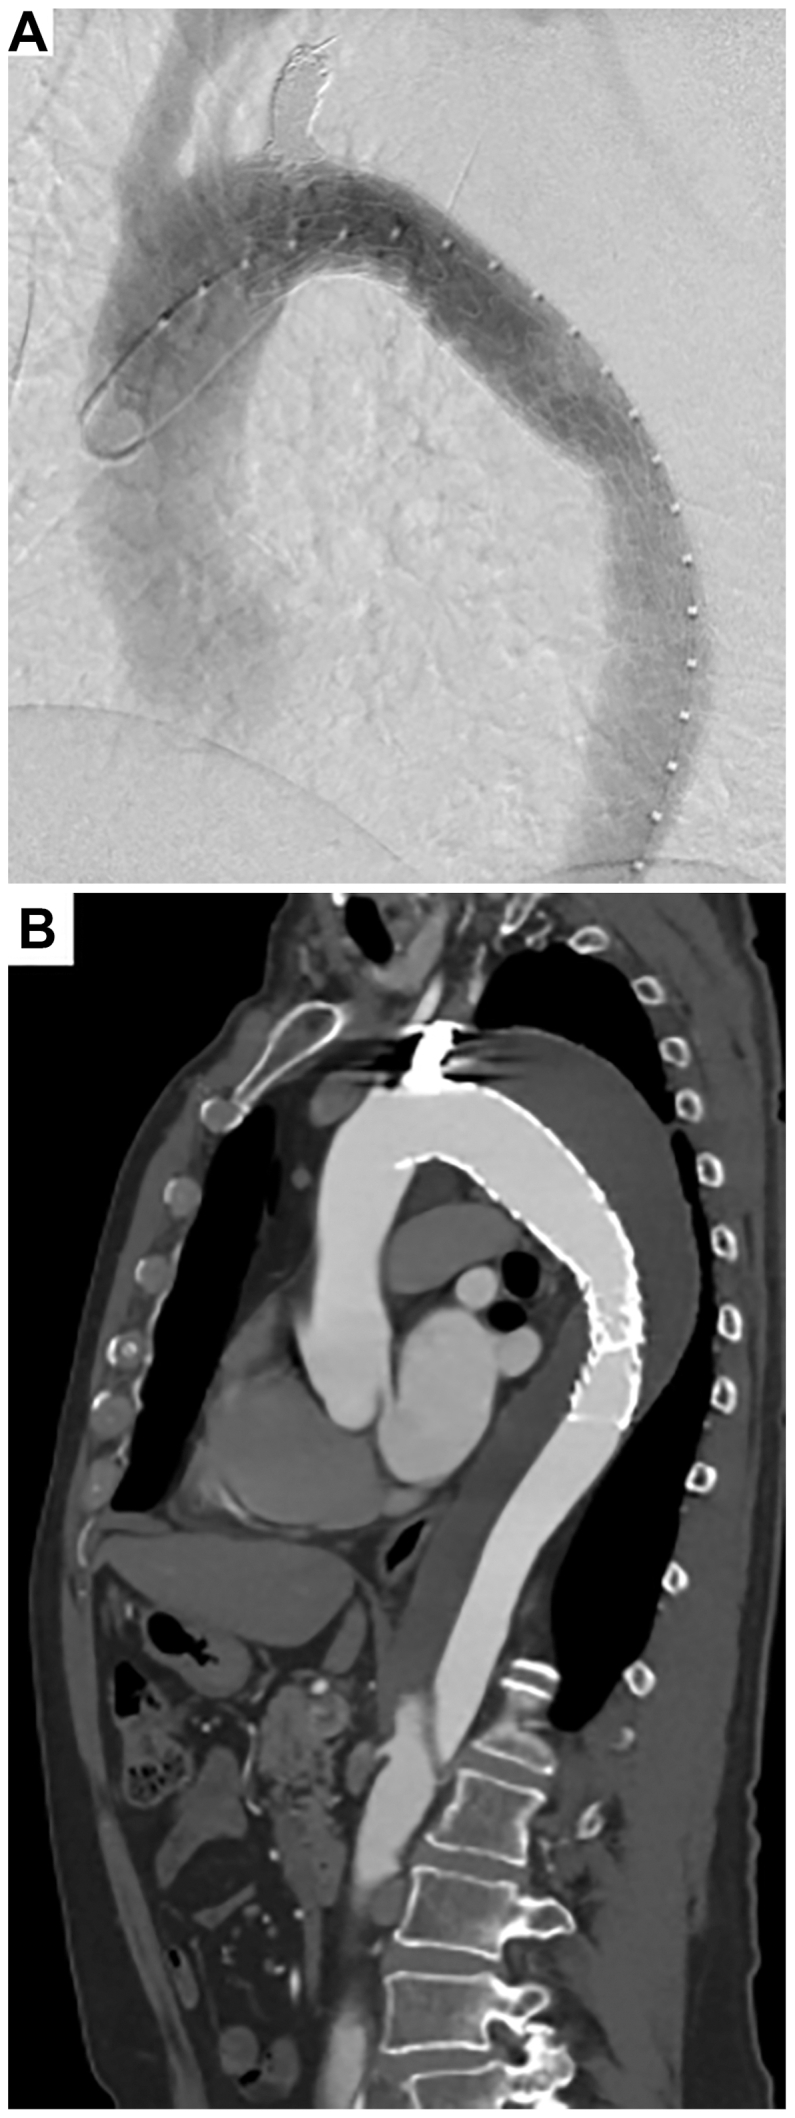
Completion angiography revealed no antegrade false lumen flow or endoleaks (A). Follow-up computed tomography (CT) angiography at 3 months demonstrated favorable aortic remodeling with complete false lumen thrombosis in the thoracic aorta (B).
